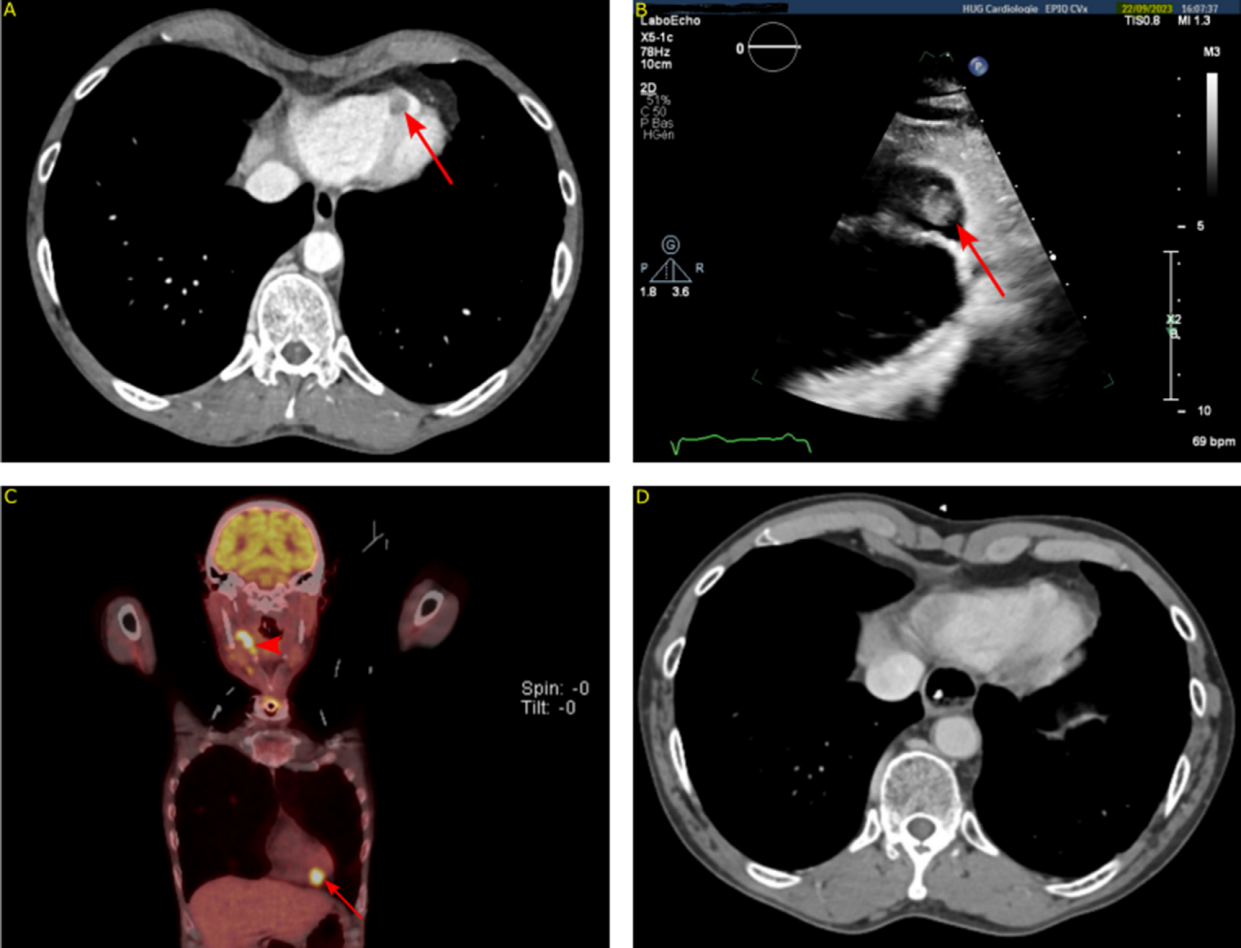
Panel A: Initial CT-scan showing an intraventricular mass (red arrow). Panel B: Echocardiography showing right ventricular lesion (red arrow). Panel C: Coronal view of initial ^18^F-FDG PET/CT showing primary cancer hypermetabolism (arrowhead) and cardiac metastasis (arrow). Panel D: Follow-up CT showing a marked regression in size of intracardiac lesion at 6 months after treatment initiation.
